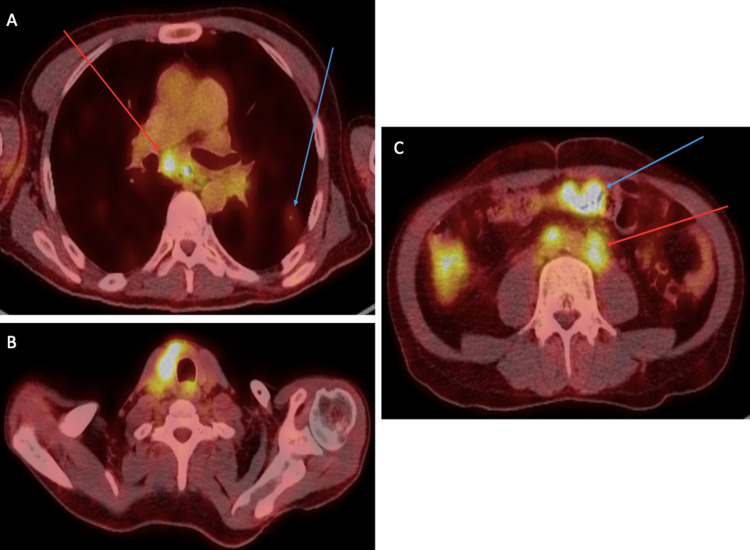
PET/CT whole body scan A) Axial-fused 18F-FDG PET/CT image showing an FDG-avid pulmonary metastasis (blue arrow) and an FDG-avid metastatic subcarinal lymph node (red arrow). B) Axial-fused 18F-FDG PET/CT image at the level of the thyroid gland showing a marked FDG-avid mass occupying the left thyroid lobe extending to the isthmus, which was likely malignant. C) Axial-fused 18F-FDG PET/CT image of the abdomen showing marked FDG-avid short segment circumferential wall thickening at the mid transverse colon (blue arrow) that was likely malignant. FDG-avid metastatic retroperitoneal lymphadenopathy was also noted (red arrow).​
